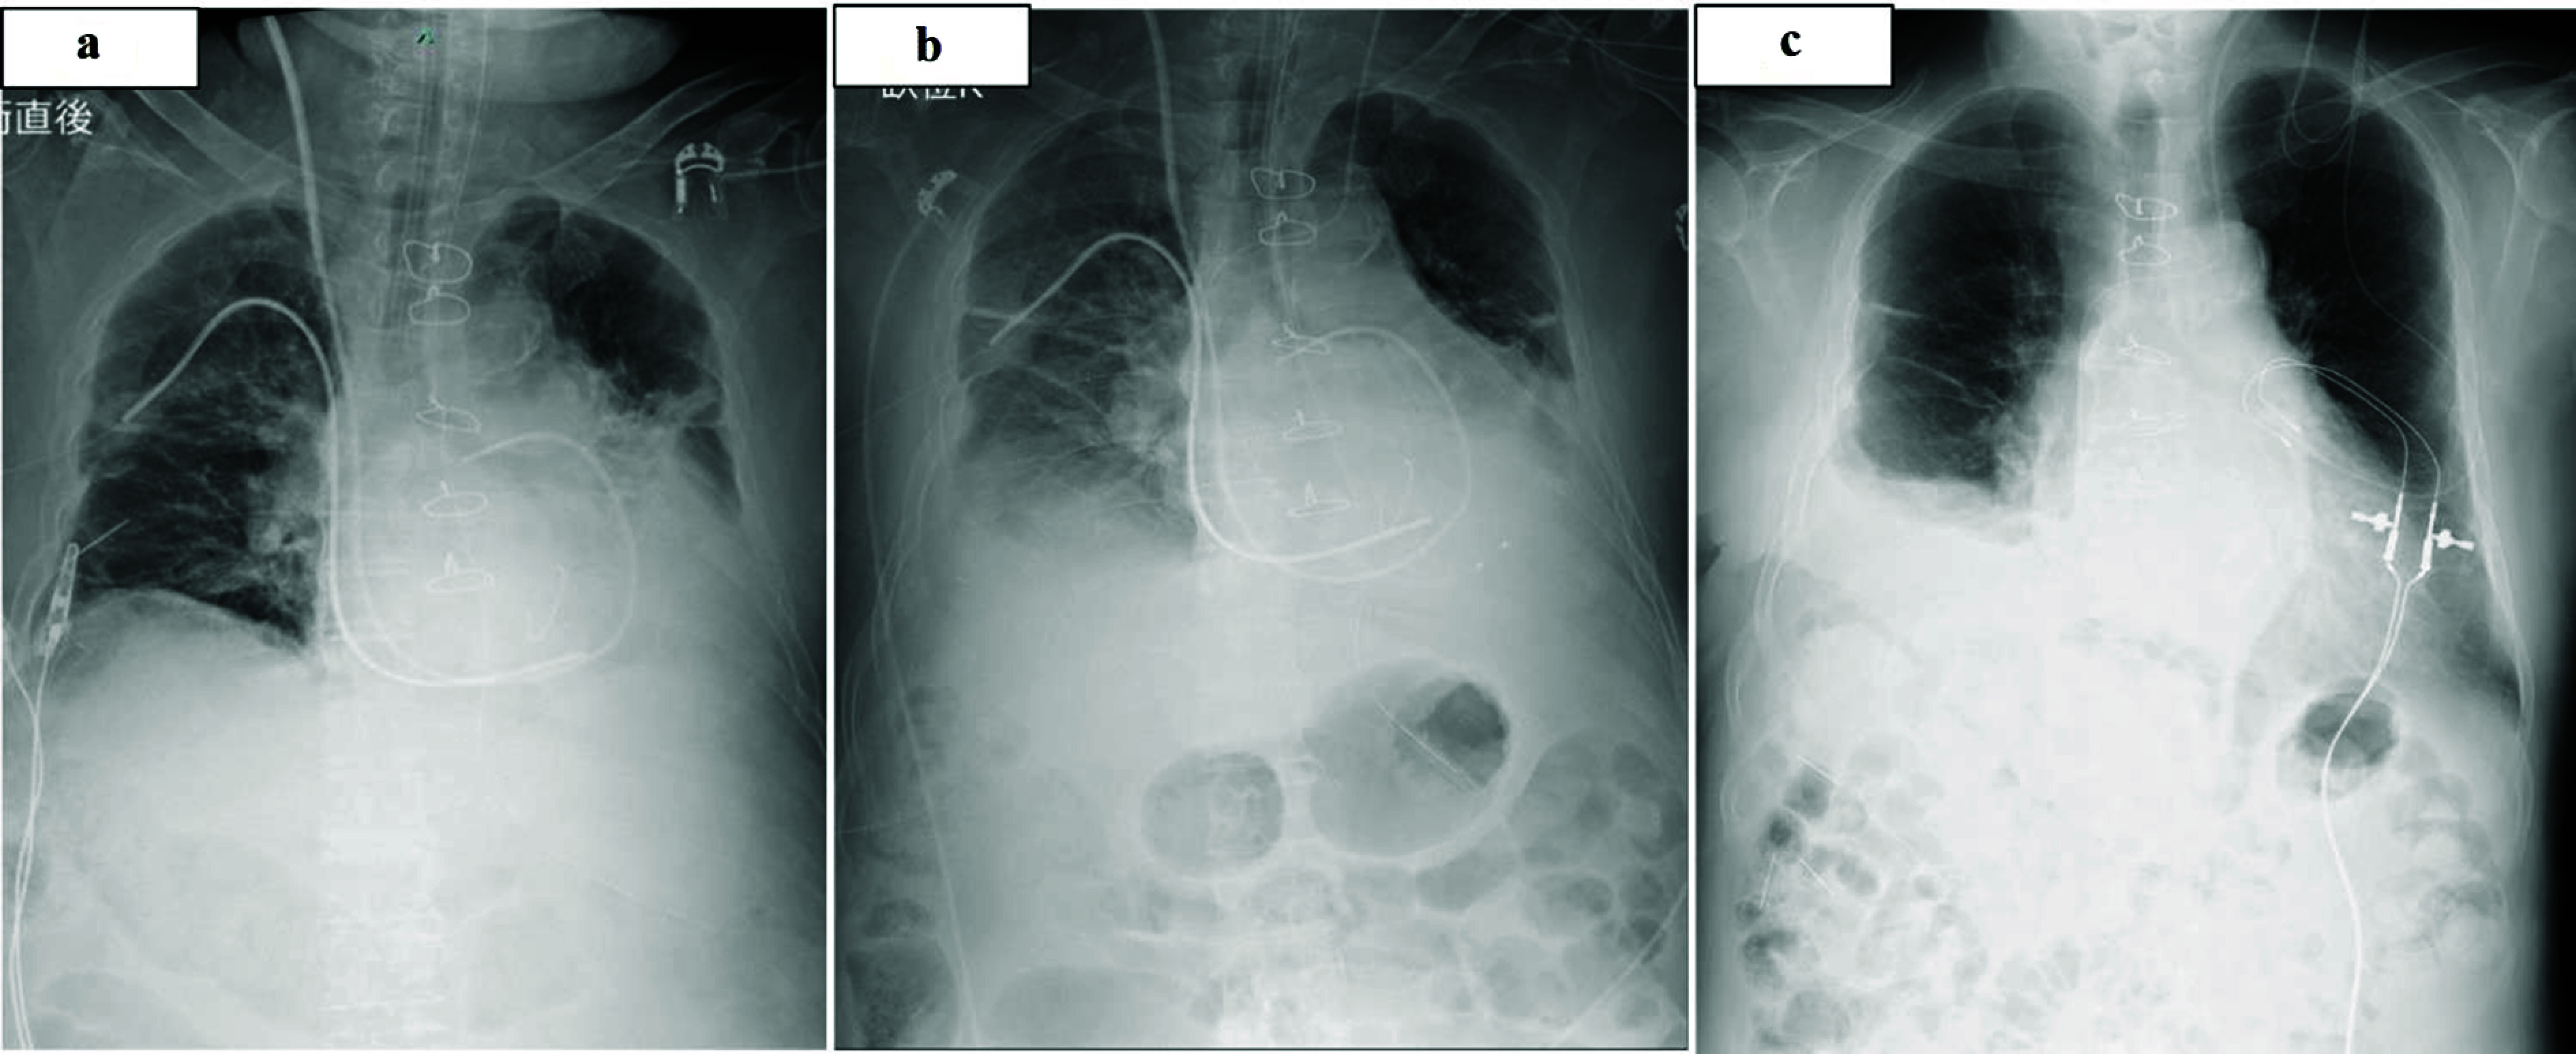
(a, b) Immediately postoperative CXR and the day after surgery CXR, which indicates overall pulmonary edema. (c) CXR indicates gradually improving from 5 days after surgery. CXR.: Chest x-ray.
